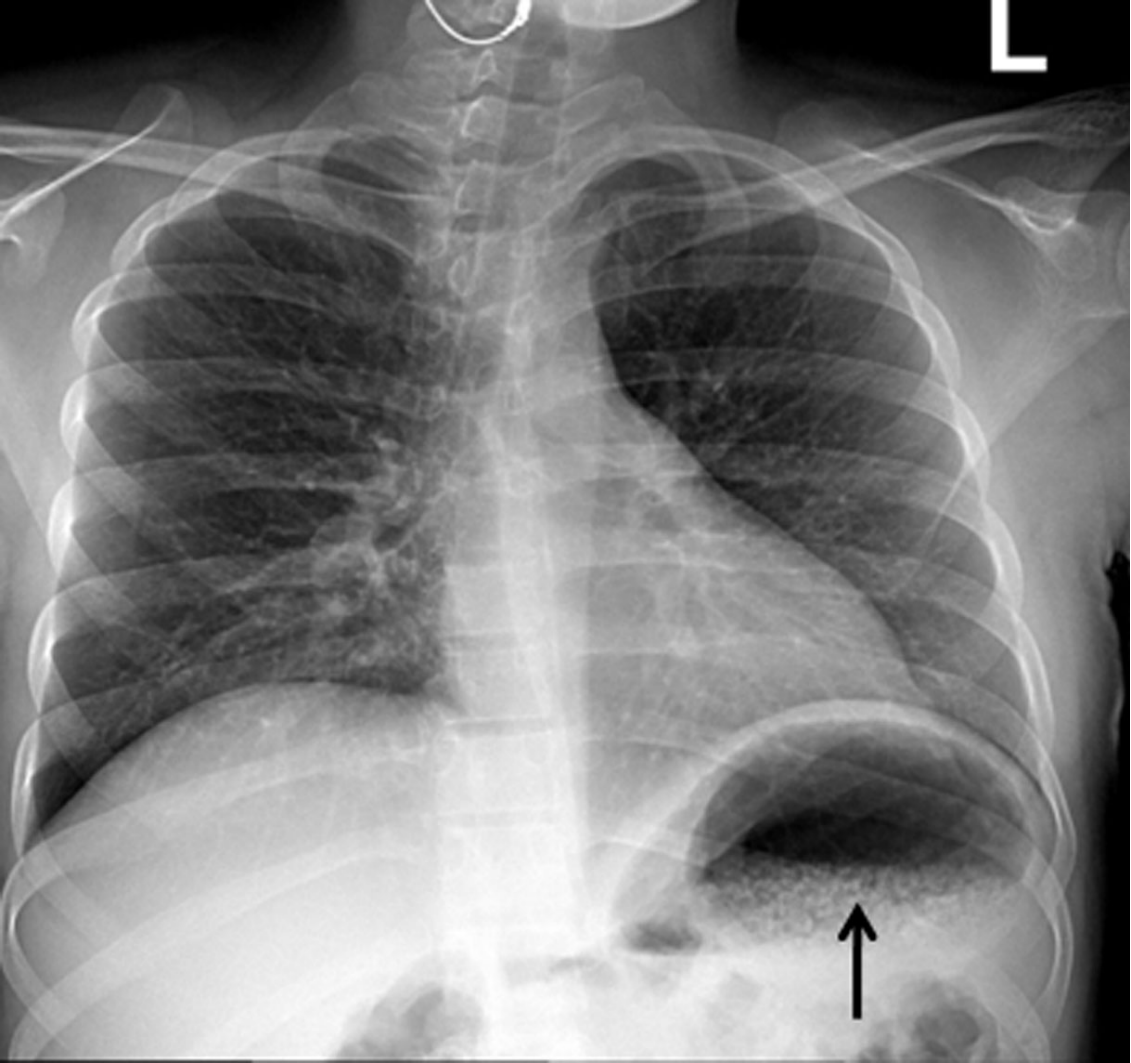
Chest roentgenogram showing innumerable bubbles outlining the stomach in a mottled distribution (arrow).
